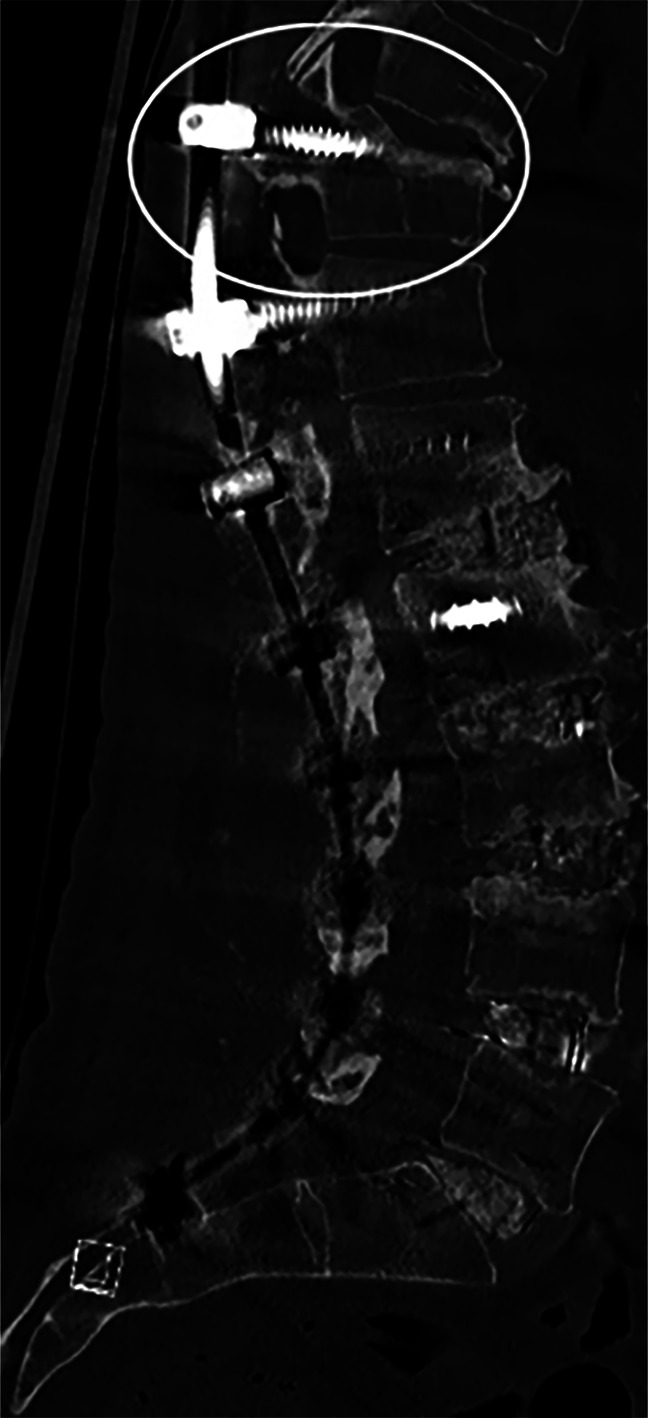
Image showing a computed tomography scan demonstrating a Chance-type fracture pattern.
